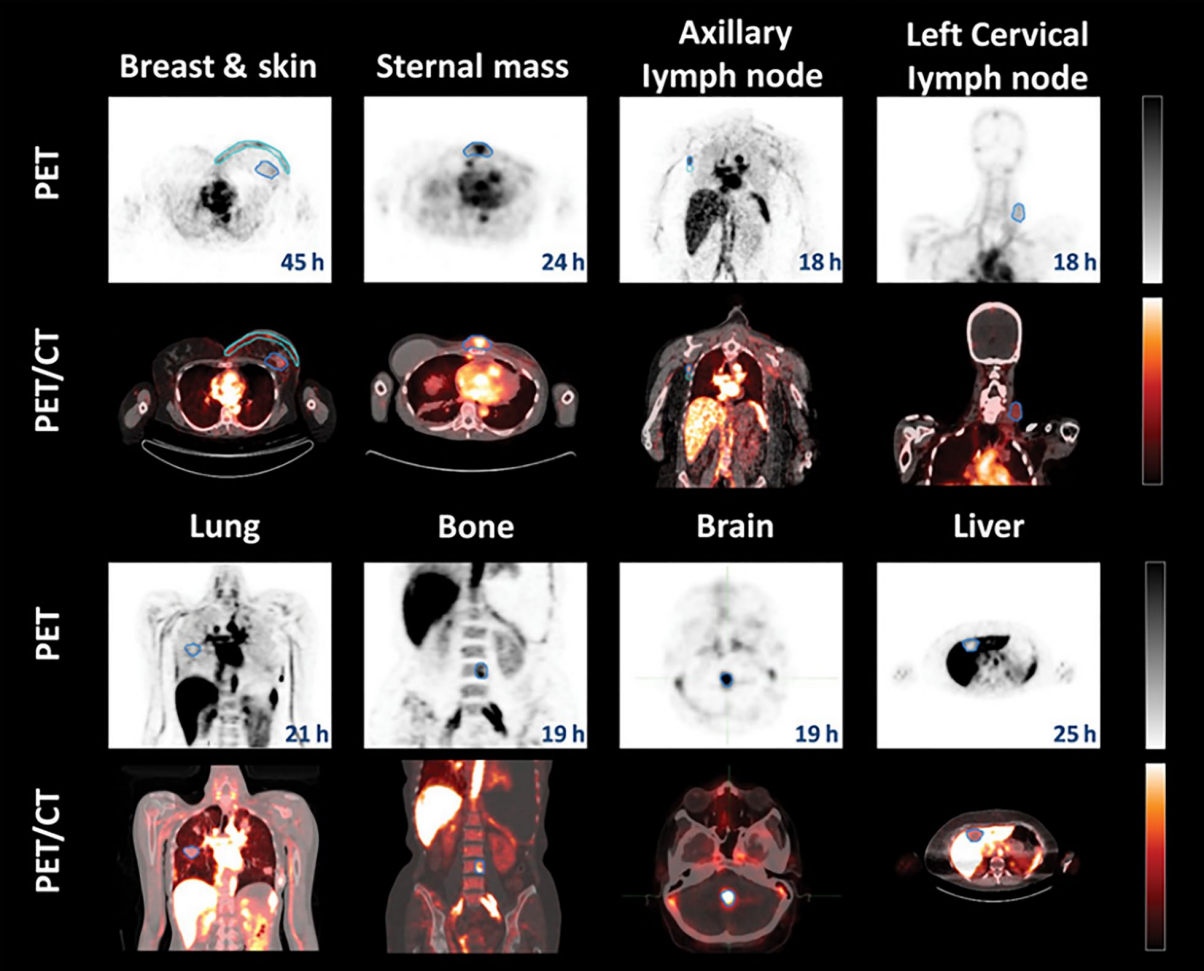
PET and PET/CT images of [^64^Cu]MM-302 in lesions at different anatomic locations. The regions of interest used to measure tumor deposition of [^64^Cu]-MM-302 are shown in blue or turquoise outlines (this figure was reproduced from Lee et al. [127] with the required copyright permission)
